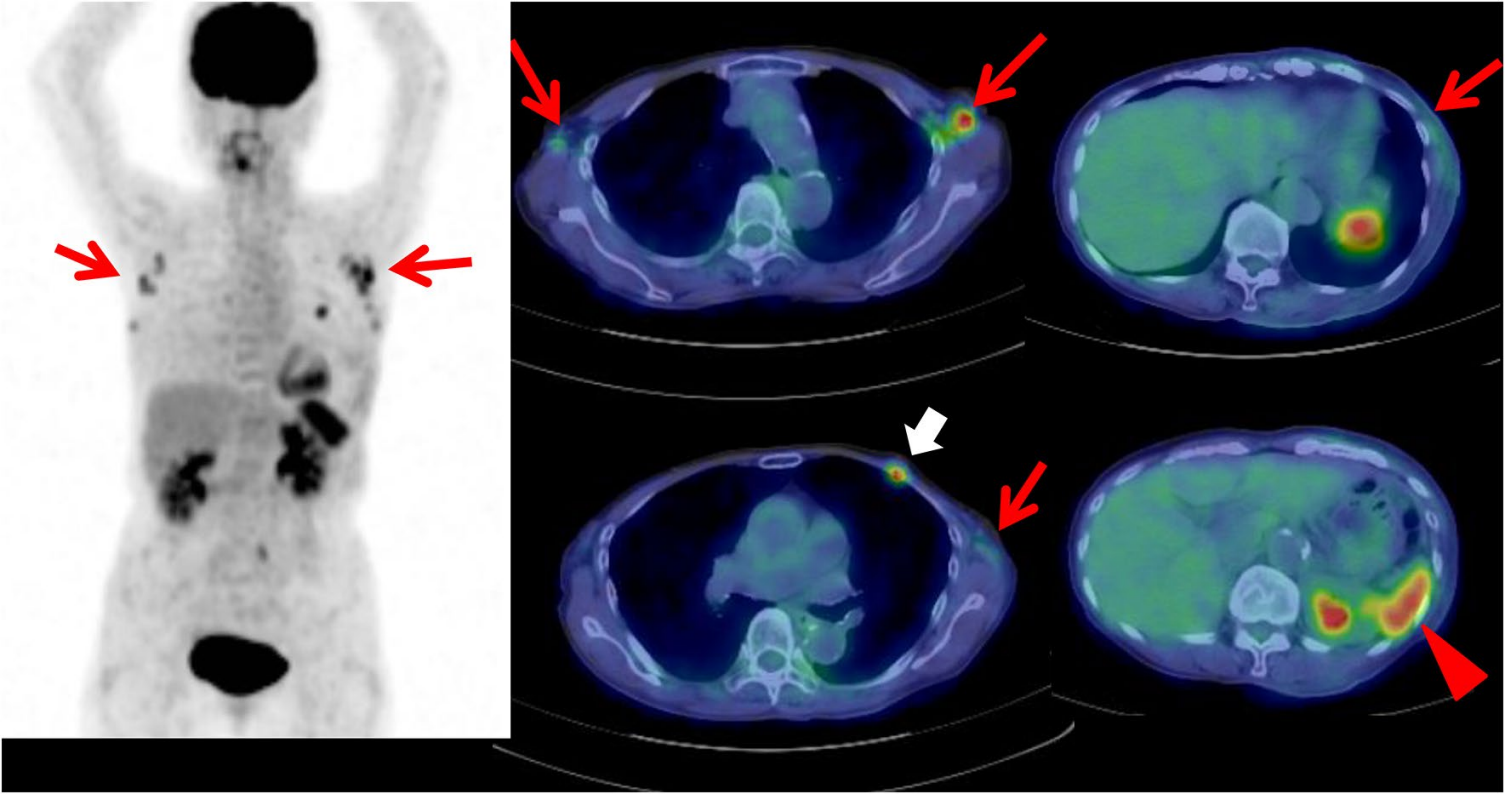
A 71-year-old female underwent FDG PET/CT scanning for left breast cancer, while she simultaneously suffered from herpes zoster lesions in left upper abdominal wall. Whole-body FDG PET maximum projection and cross-sectional images showed FDG uptake (SUVmax 1.9) in the active herpes zoster lesions in left abdomino-chest wall skin (arrows) and FDG uptake (SUVmax 4.8) in bilateral axillary lymph nodes (arrows), with FDG uptake (SUVmax4.7) in left breast cancer (white arrow). Diffusely increased FDG uptake (SUVmax5.6) was also seen in the spleen (arrowhead), which was probably associated with active herpes zoster. While FDG-avid lymph nodes of the left axillary region mimicked lymph node metastasis, the surgery of breast cancer after regression of active herpes zoster revealed the absence of metastasis in these lymph nodes
